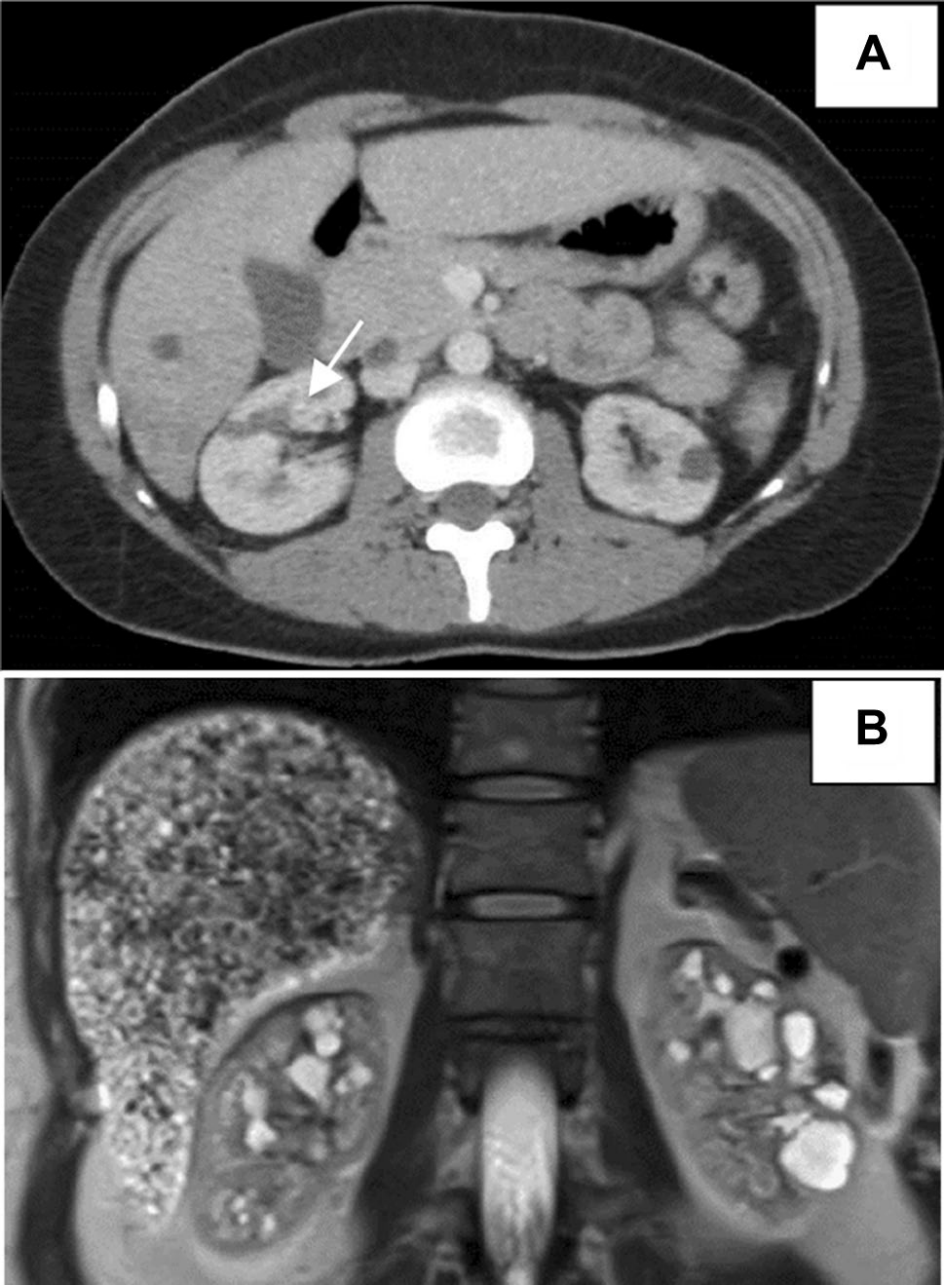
A Upper: axial computed tomography showing hypointense cysts of variable size in both kidneys and throughout the hepatic parenchyma. Microlithiasis (arrow) B Lower: T2-weighted magnetic resonance imaging: normal-sized kidneys with bilateral cysts located mainly at the cortico-medullary junction level and enlarged and heterogeneous liver containing multiple millimetric hyperintense lesions
